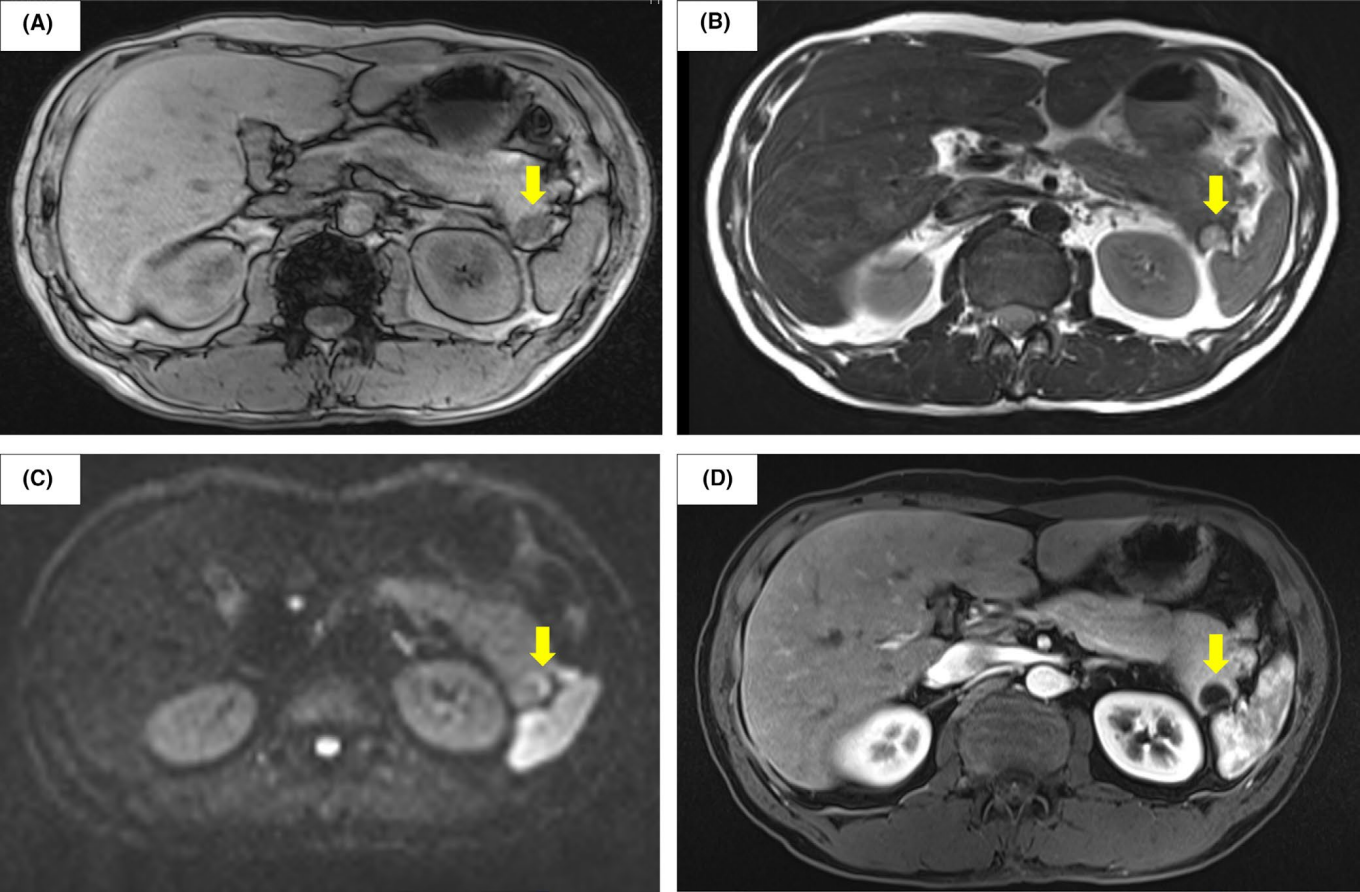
Magnetic resonance imaging (MRI): A, The T1‐weighted image shows a cystic lesion measuring 18 × 14 mm with low signal intensity (arrow). B, The same lesion has a high signal intensity on a T2‐weighted image (arrow). C, Part of the cyst wall was hyperintense (arrow) on diffusion‐weighted imaging. D, Early uptake of the dye by this part of the wall (arrow) on dynamic MRI is shown
